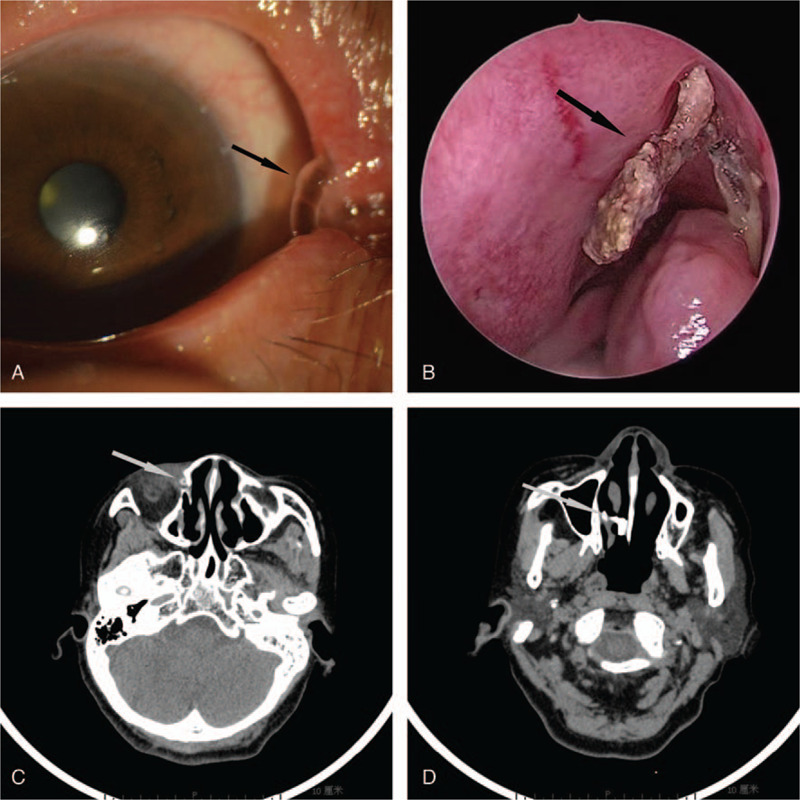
Clinical photographs of the puncta (A), including a view of the inferior nasal meatus using nasal endoscopy (B) and CT scan (C, D). The low density in the center of the silicone tube indicates that the tube is unobstructed (C), but the high density calcification can be seen at the end (B, D). Proper insertion of a silicone tube is shown (arrows). CT = computed tomography.
